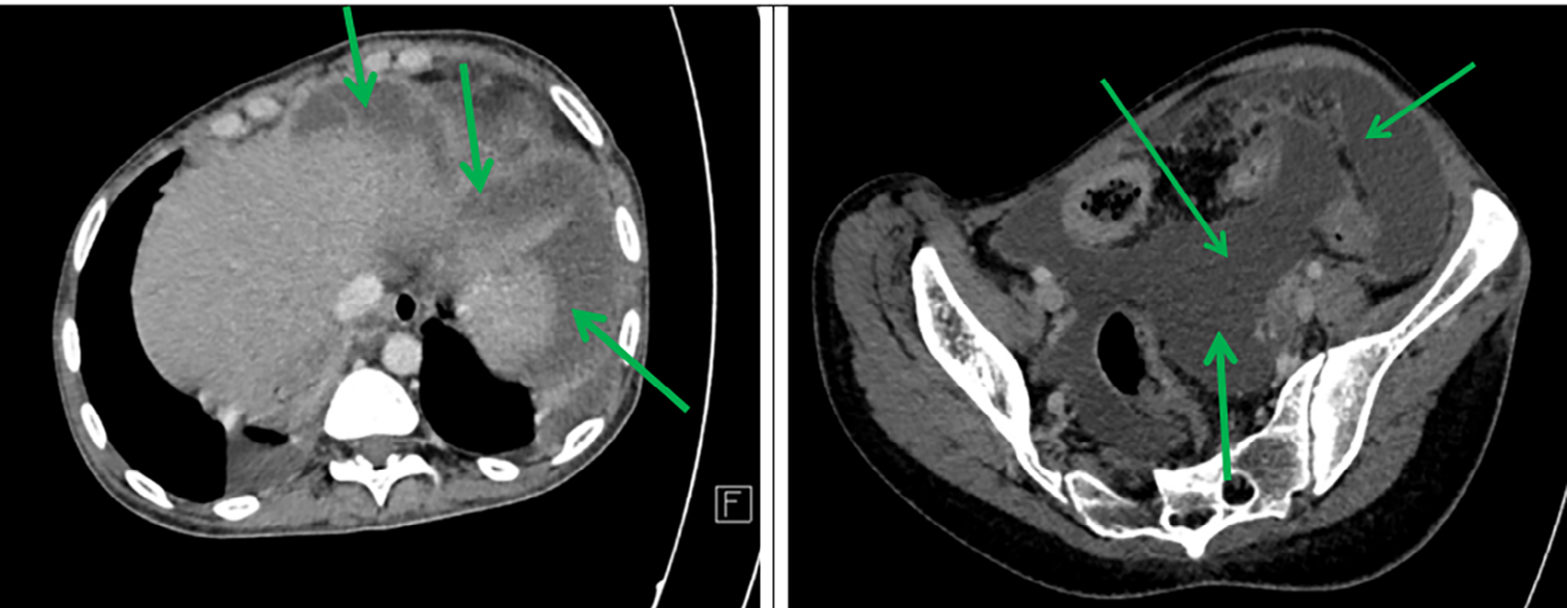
Axial contrast-enhanced abdominal CT scan after contrast injection showing abundant peritoneal effusion (green arrows).
